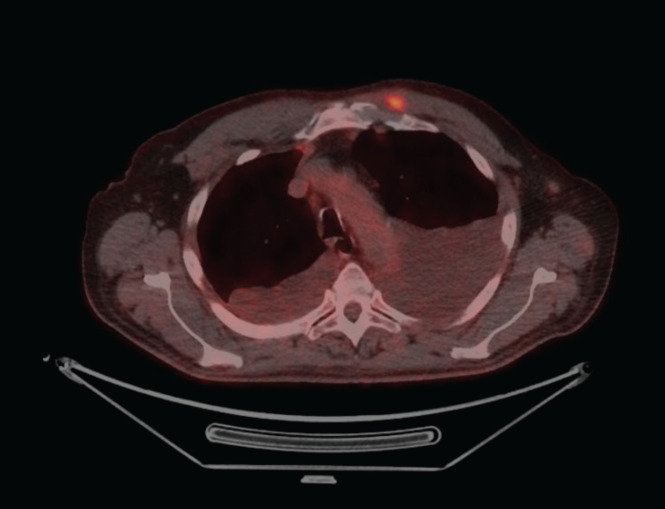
PET- CT with FDG uptake in a metastasis of the left pectoral muscle.
